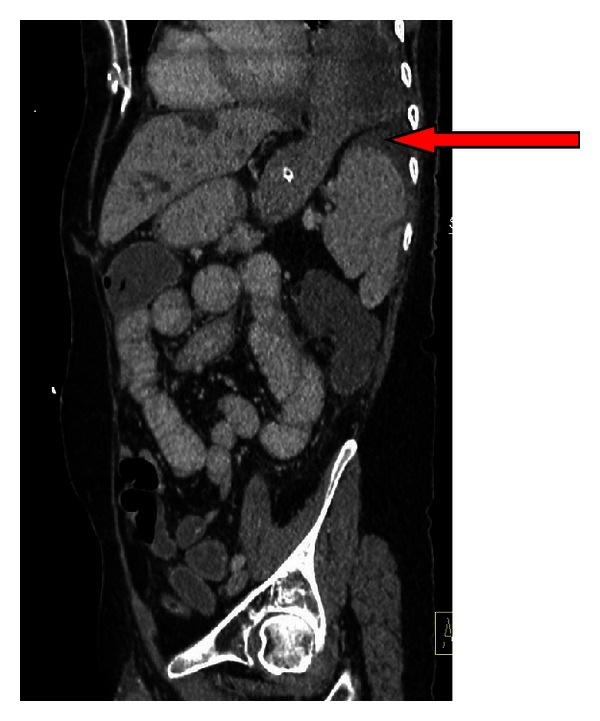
CT Case 1; saggittal section showing herniation of the gastric fundus through a diaphragmatic defect (as indicated by arrow) with evidence of incarceration-note nasogastric decompression of the abdominal portion of the stomach in the presence of a dilated nondecompressed thoracic stomach.
